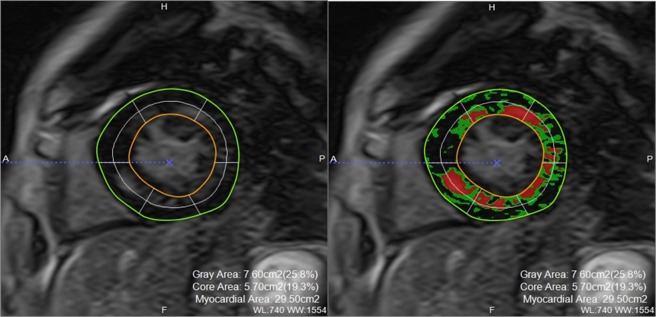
Measurement of LGE by MRI. LGEcore, LGEgray, and LGEcore+gray were calculated as areas with the above-threshold signal intensity in the ROI (≥5 SD for LGEcore and 2–5 SD for LGEgray compared to the normal area). ROI: region of interest. LGE: late gadolinium enhancement.
